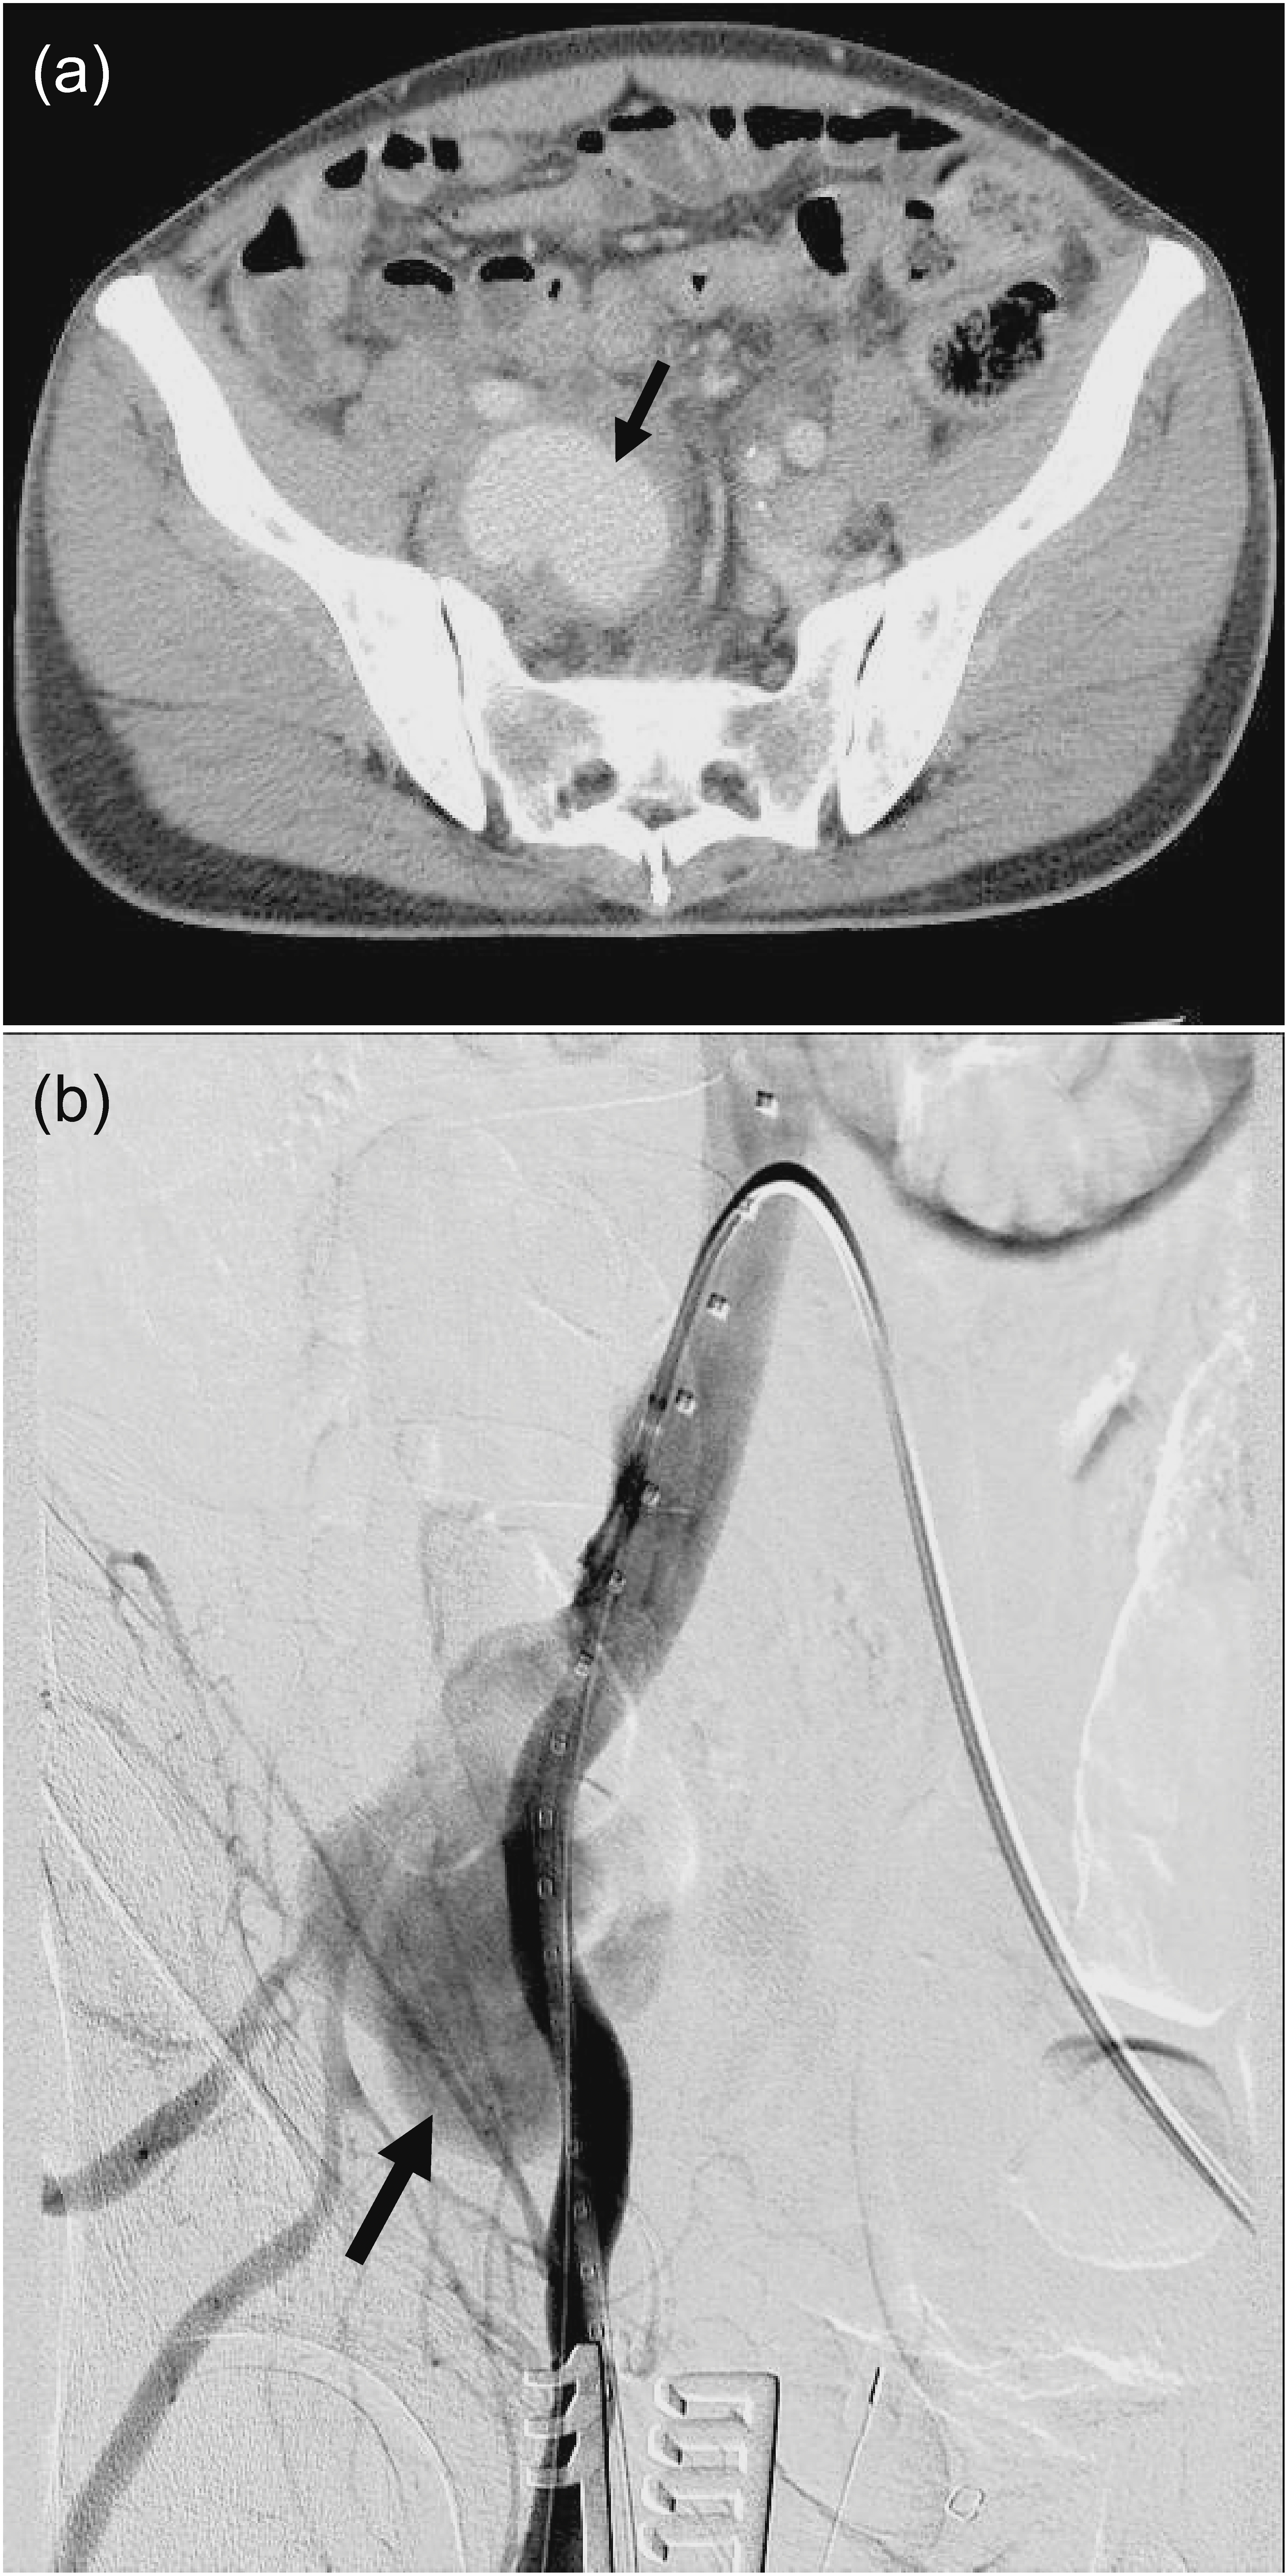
Fig. 1 (A) Abdominal contrast-enhanced computed tomography showing a pseudoaneurysm (60×45 mm) in the right internal iliac artery (arrow). (B) Contrast imaging of the right common iliac artery (pretreatment) showing a pseudoaneurysm in the right internal iliac artery (arrow), similar to the abdominal computed tomography finding.
